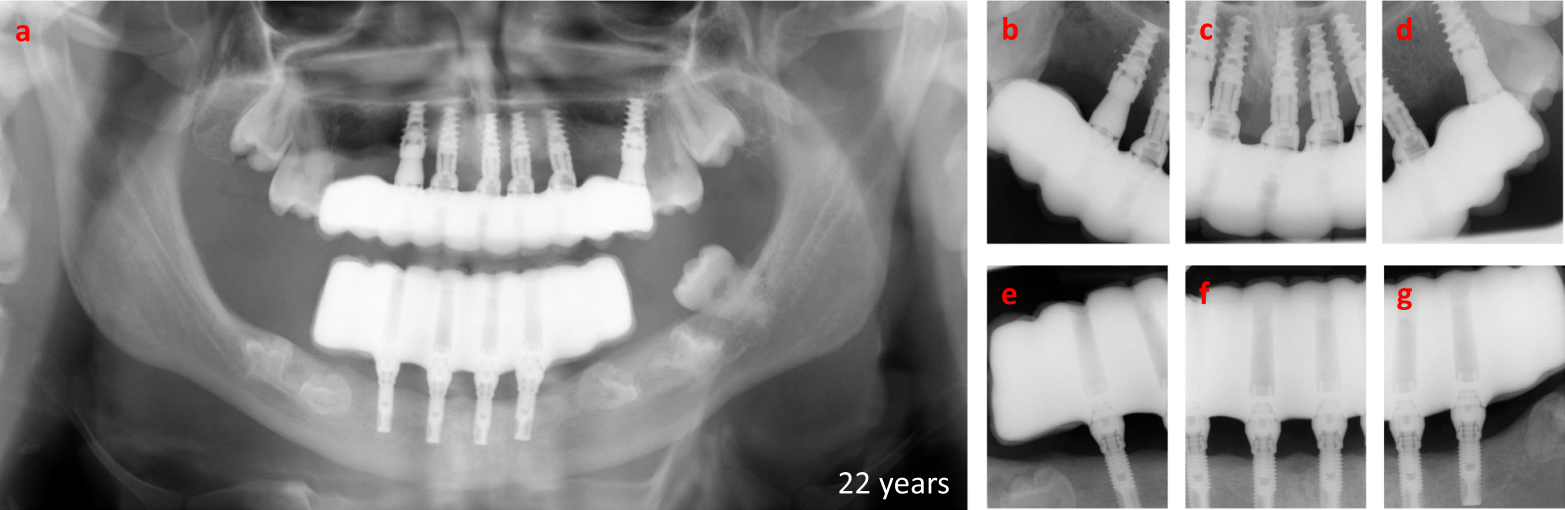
Radiographic overviews three-years post-loading of the implant-fixed bridges at the age of 22 years. a radiographic panoramic view, b-d intraoral radiographs of the upper jaw, and e–g intraoral radiographs of the lower jaw
